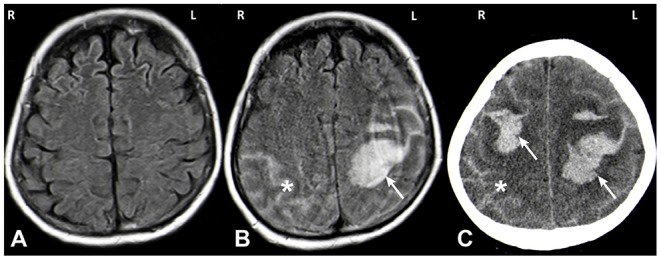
MRI (A,B) and CT (C) images. MRI imaging on day of admission after a first seizure was unremarkable (A: axial FLAIR). MRI on day 5 showed a spontaneous left hemispheric ICH with a subarachnoid component (SAB) (B: axial FLAIR; white arrow: ICH, asterisk: SAB). One day later the patient deteriorated again and CT imaging showed a right sided sICH and edema of the left hemisphere (C: axial CT; white arrow: ICH, asterisk: SAB).
